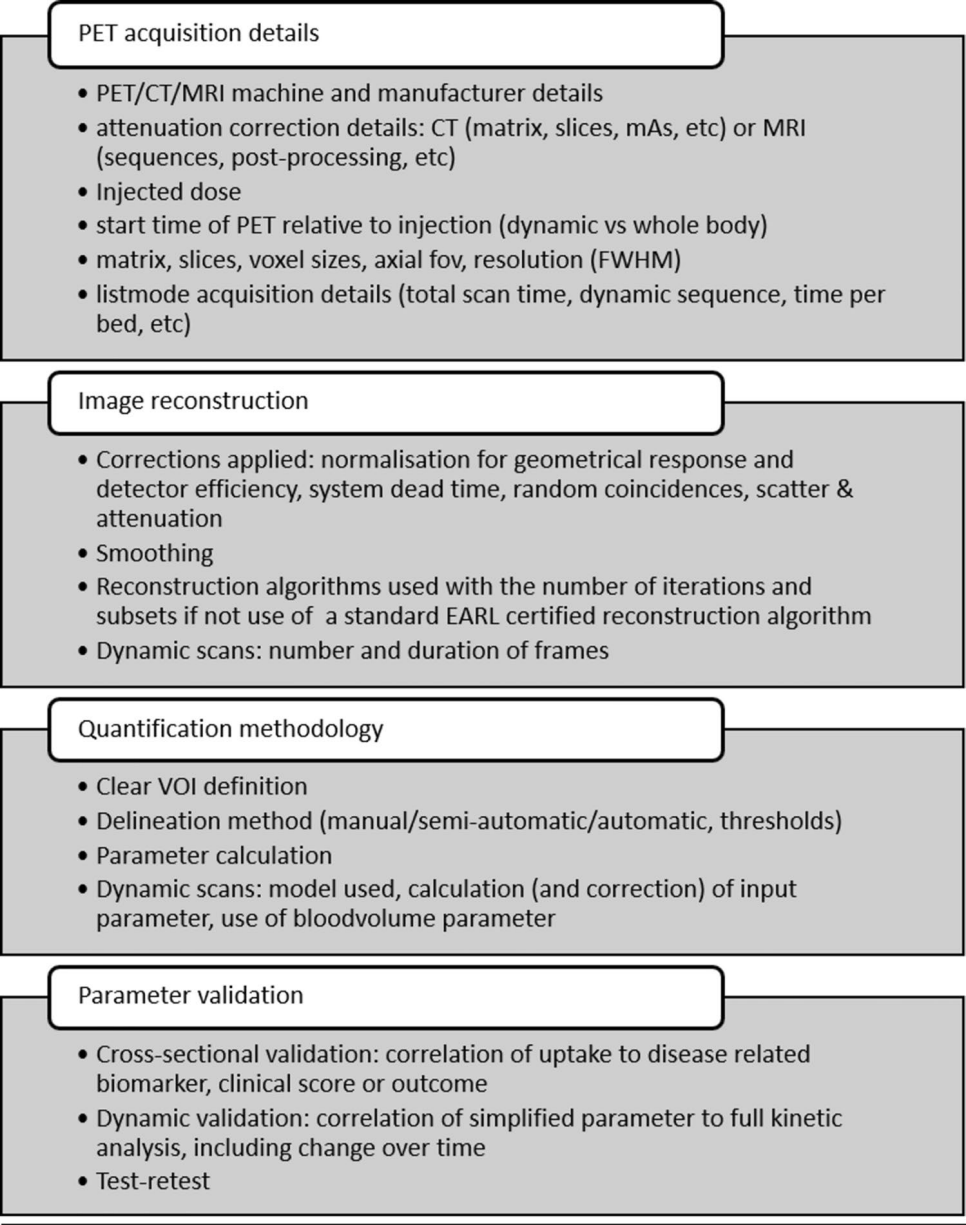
Information to be considered for documentation to reliably reproduce the quantification of an uptake parameter obtained with the [18F]NaF PET, PET/CT or PET/MRI
